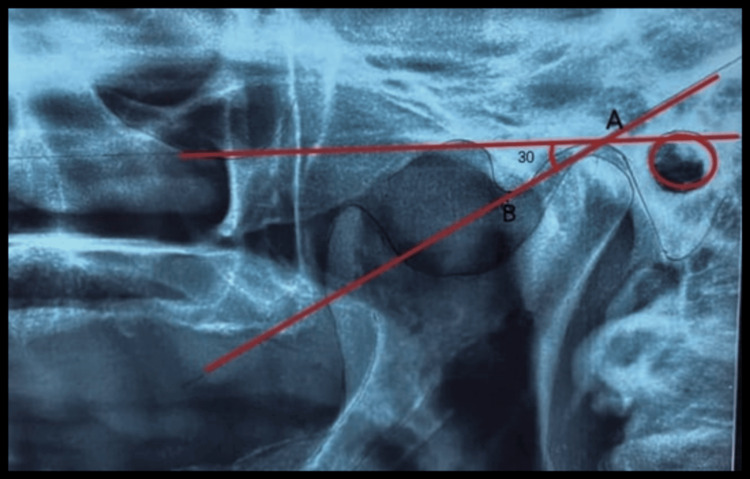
Tracing on OPG. Point A is the most superior point of curvature, and Point B is the most inferior point of curvature. The horizontal line represents the FH plane. Angle measured is 30°. OPG: Orthopantomogram, FH: Frankfort Horizontal Plane
